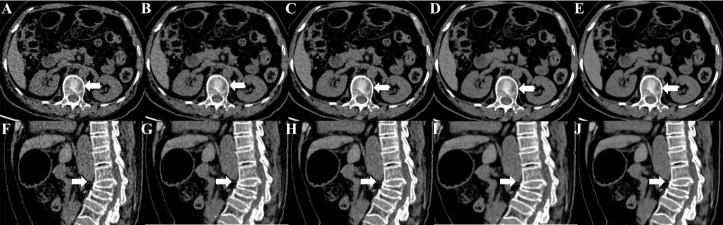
Unenhanced CT images of a 67-year-old female for osteoporotic vertebral fracture in the L3 vertebrae. CT images were reconstructed with FBP (A, F), ASiR-V50% (B, G), DLIR-L (C, H), DLIR-M (D, I) and DLIR-H (E, J) with a slice thickness of 1.25mm at 0.75 mGy. The L3 vertebrae body was shown as a severe collapse in sagittal images (arrow), and the vertebral compression appearance was presented in axial images (arrow). The BMD values of FBP, ASiR-V50%, DLIR-L, DLIR-M and DLIR-H were 72.49, 72.74, 71.68, 70.11 and 69.24 mg/cm^3^ for L1 vertebrae, 67.33, 69.11, 70.25, 65.38, 68.49 mg/cm^3^ for L2 vertebrae, 62.08, 45.92, 49.57, 52.21, 50.93mg/cm^3^ for L3 vertebrae, respectively. CT, computed tomography; FBP, filtered back projection; ASiR-V50%, adaptive statistical iterative reconstruction-veo 50%; DLIR(L/M/H), deep-learning image reconstruction, level low, medium, and high; mGy, milligray; BMD, bone mineral density.
